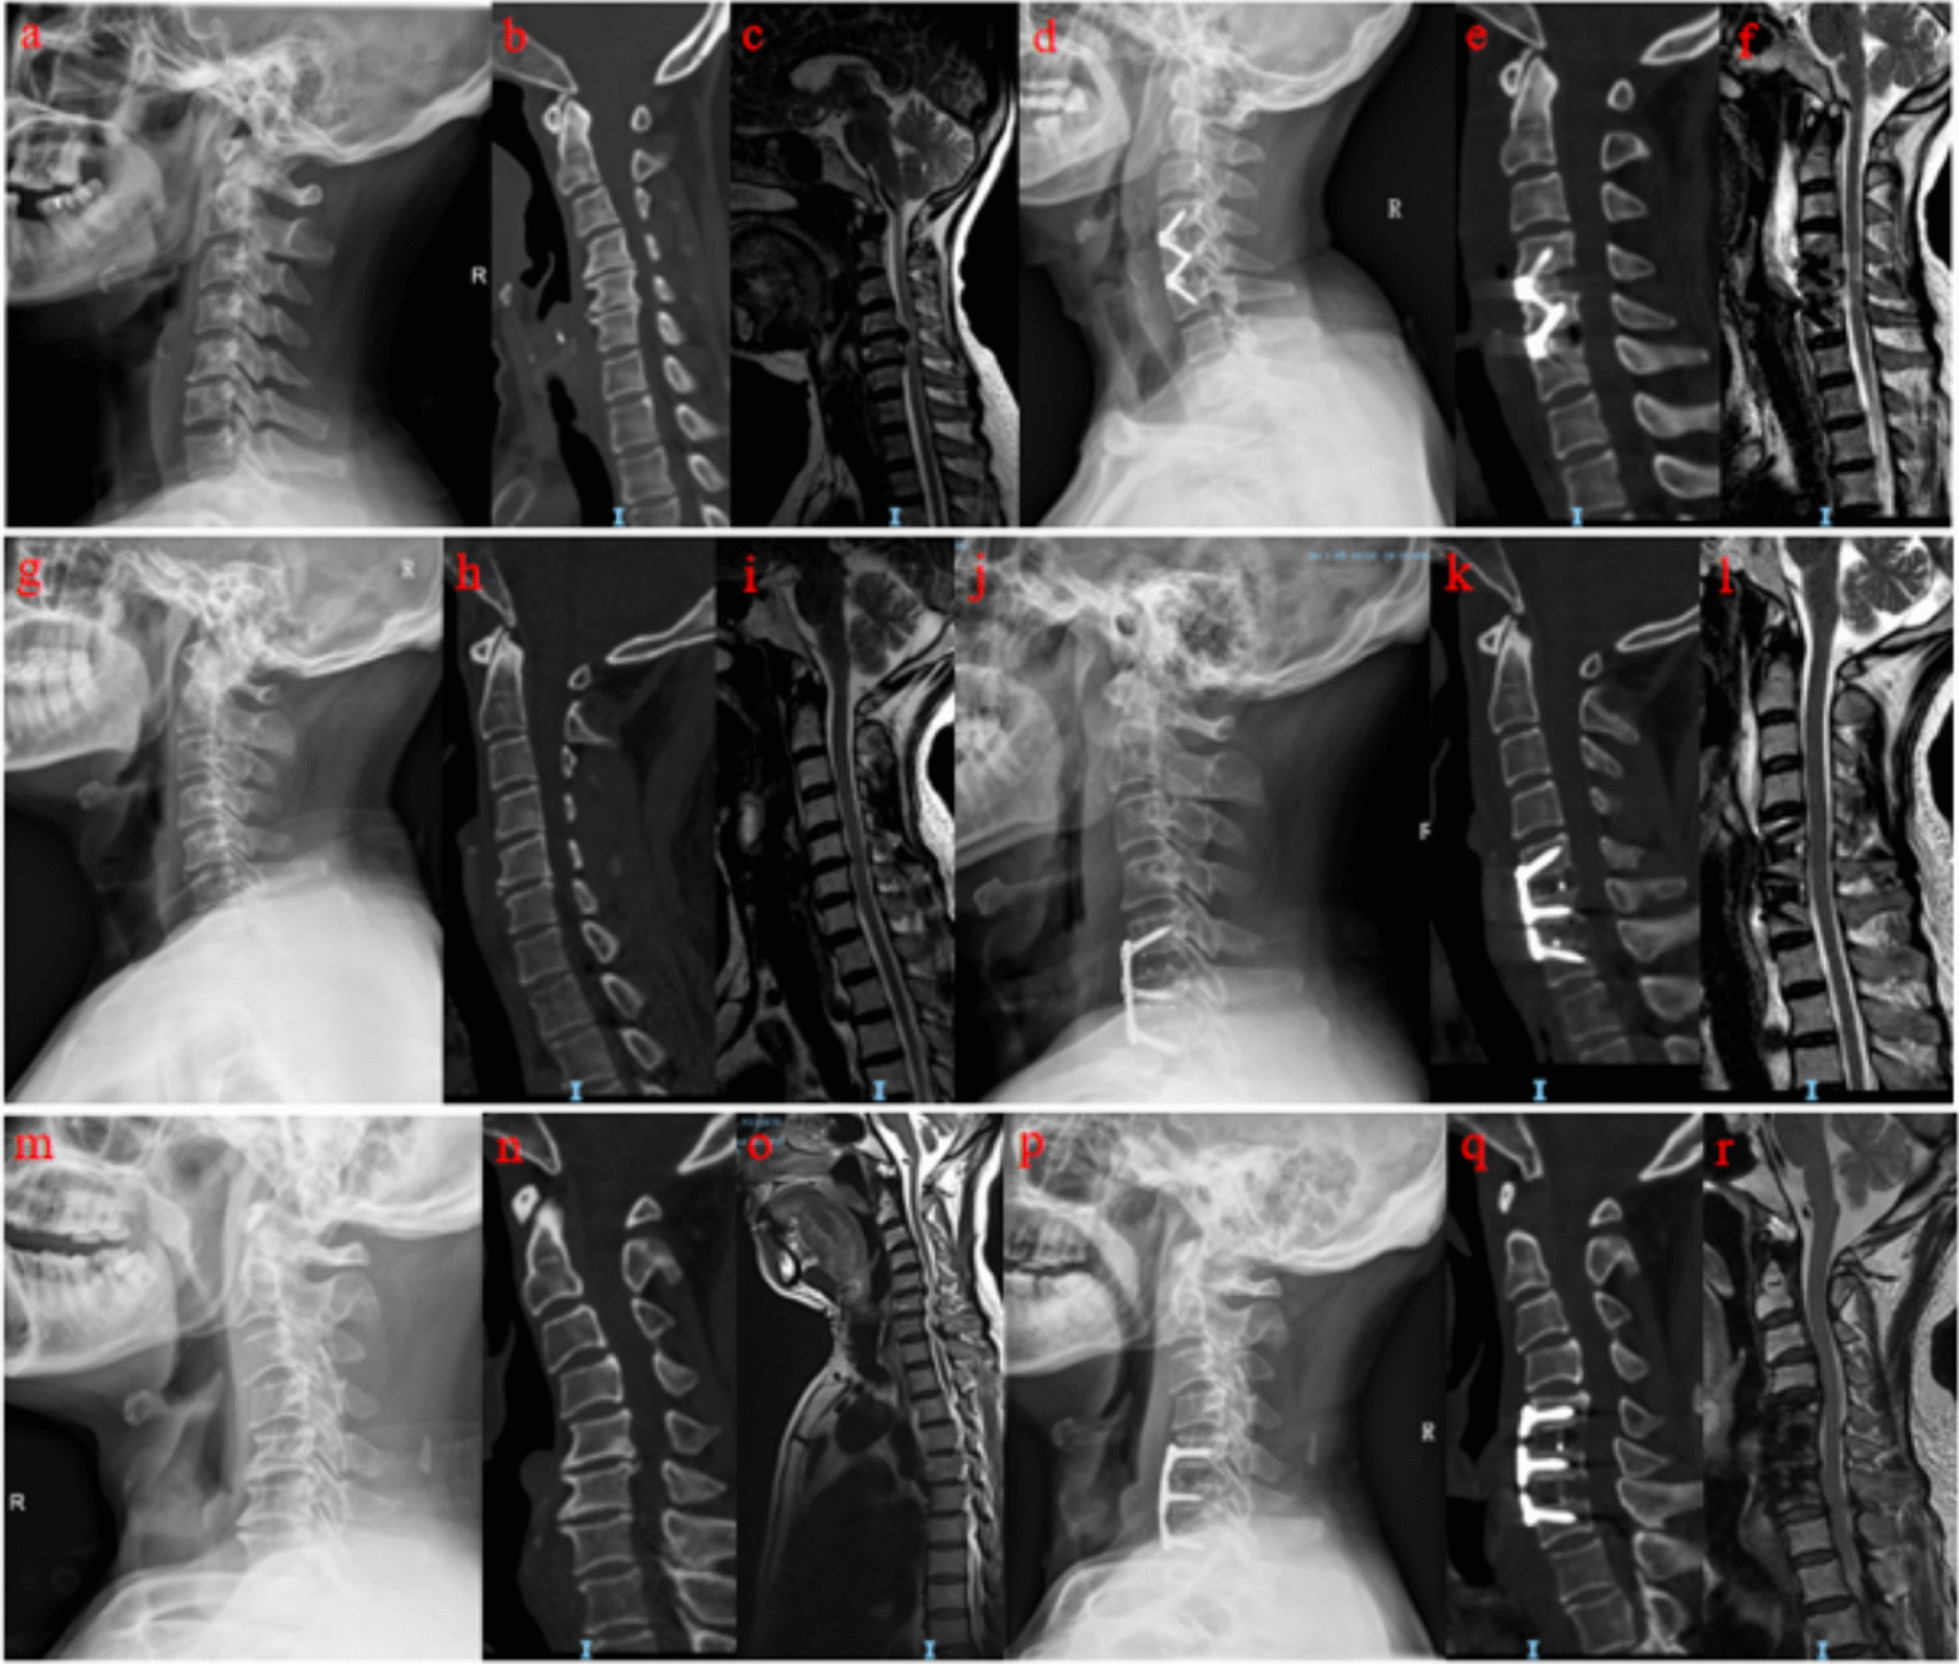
a–f: Pre- and postoperative radiographs of a 58-year-old woman following C4–C6 ACDF with the Zero-P system showing significant spinal improvement and decompression. g–l Post-C5-7 ACDF with the ZEVO System. Imaging of a 51-year-old man revealed resolved herniation and the absence of significant stenosis postsurgery. m–r: The efficacy of the Skyline System was demonstrated in a 71-year-old man, with pre- and postoperative images showing corrected alignment and decompression at C5–C7
